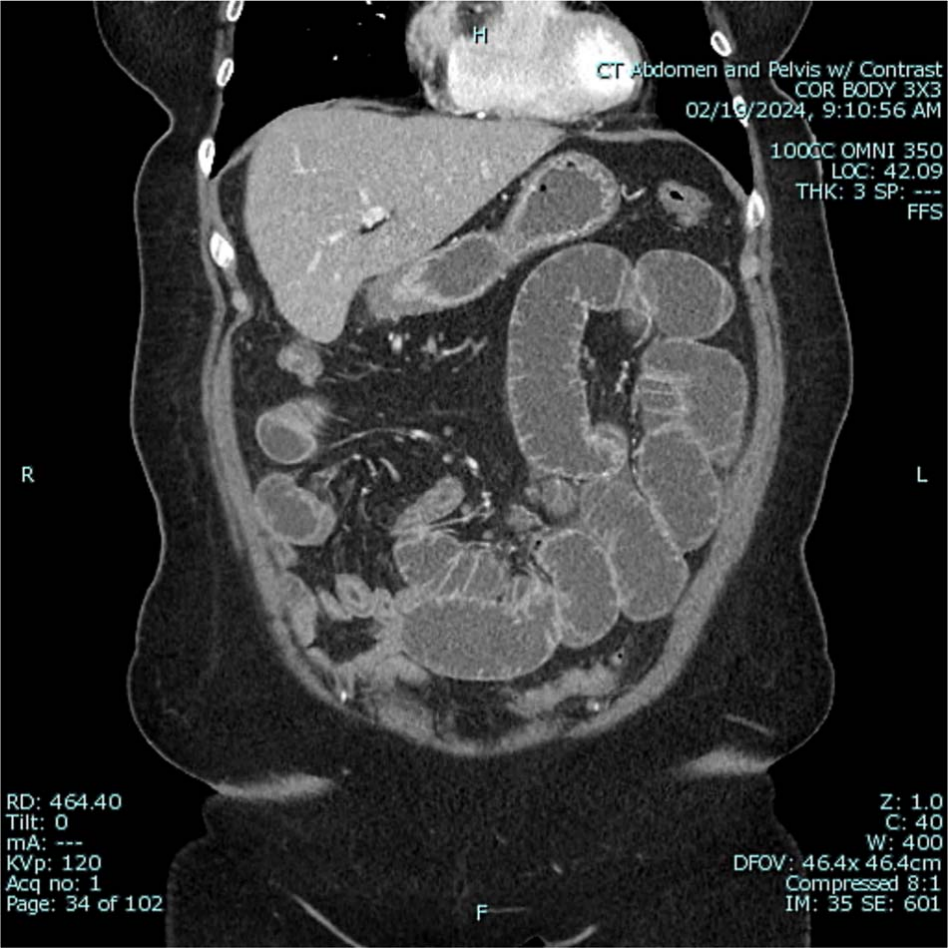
Preoperative CT of abdomen in coronal view, demonstrating small bowel obstruction.
